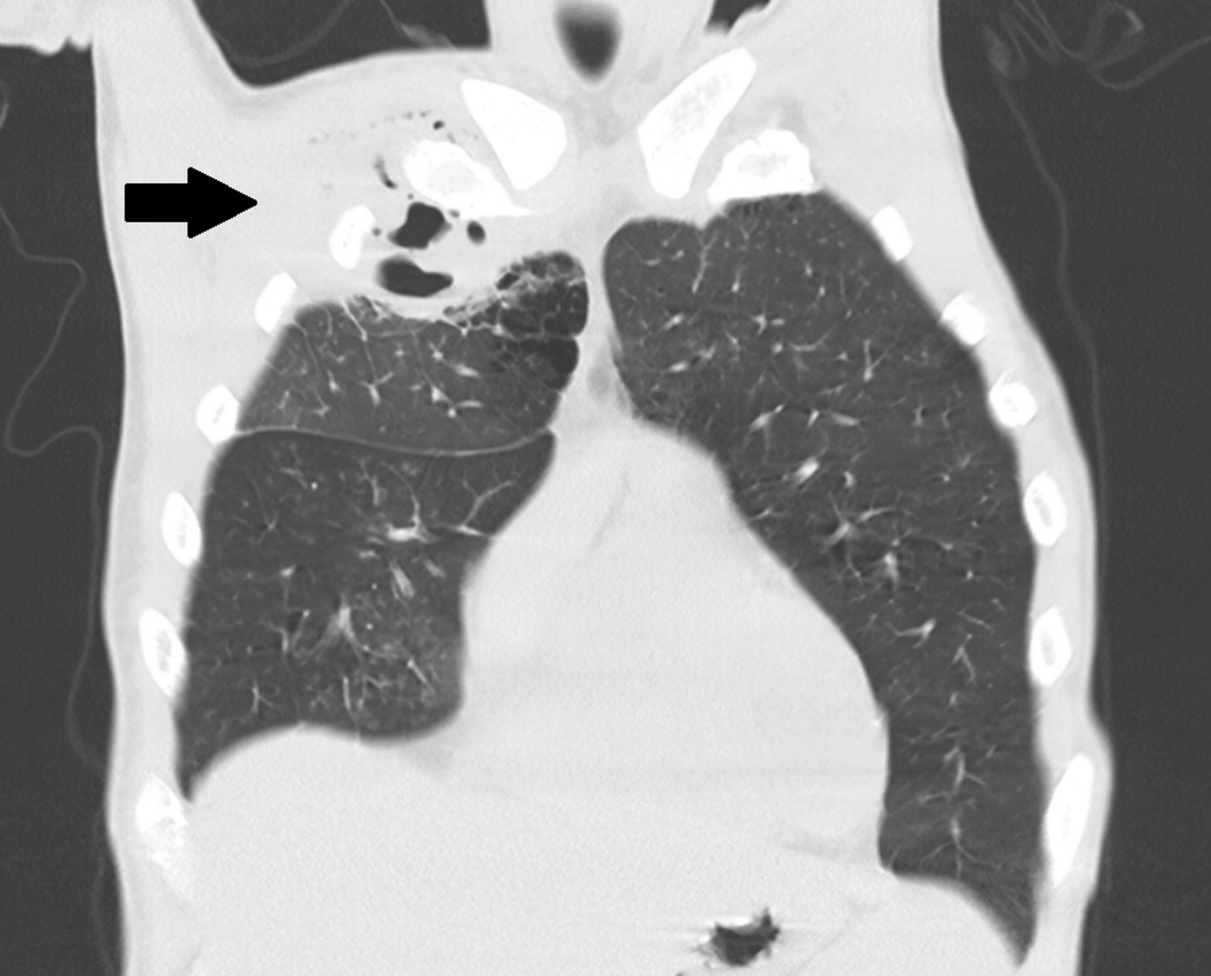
Coronal contrast-enhanced chest CT (lung window) demonstrating a large multiloculated gas-containing abscess in the right upper lobe, with loss of the normal plane between the lung and the anterior chest wall, consistent with trans-thoracic extension.
